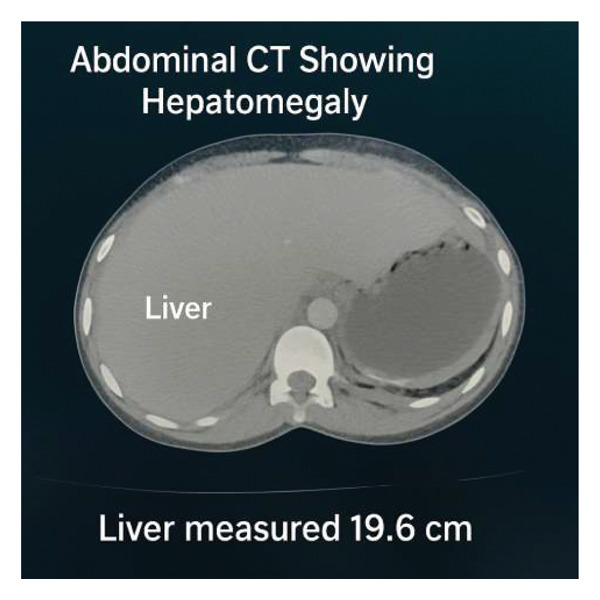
Abdominal computed tomography scan demonstrating hepatomegaly.
